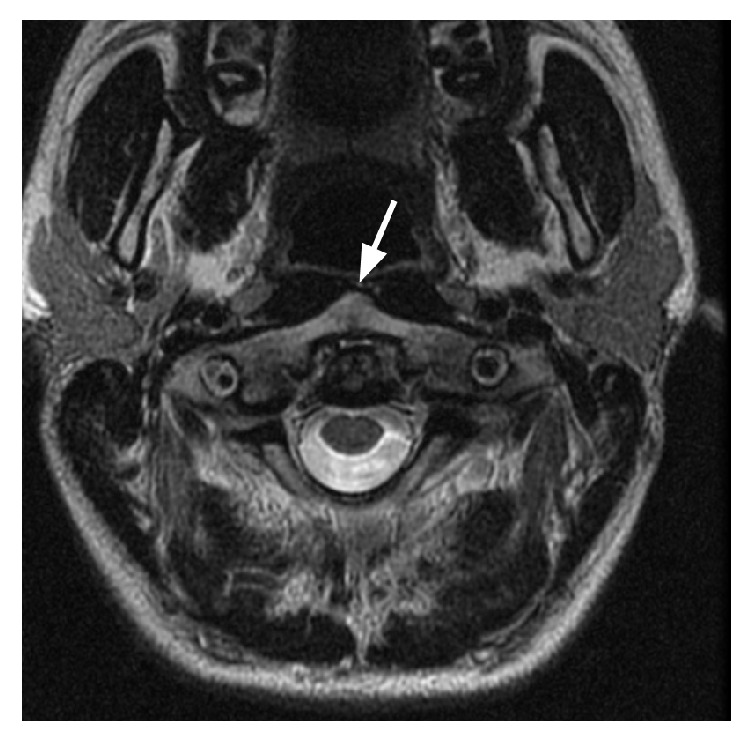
Cervical MRI image showing mild degenerative changes of the atlantoaxial junction (white arrow).
